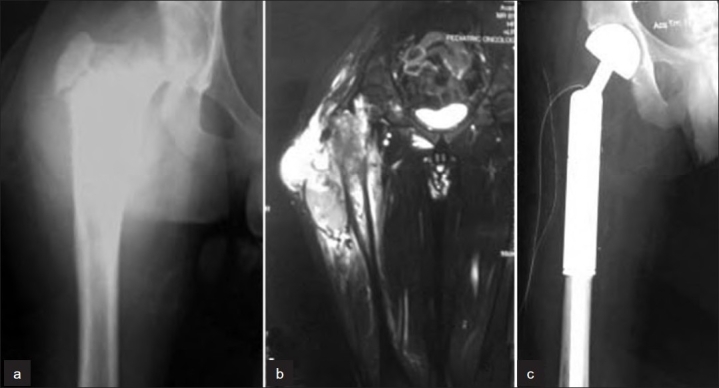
Ewing's sarcoma of the right proximal femur in a 15 yr old male, Enneking stage IIB. (a) X-ray of hip (anteroposterior view) showing the bony destruction with soft tissue mass. T2W1 saggital of hip (b) showing the intramedullary extent of the proximal femur involved along with extracompartmental extension with soft tissue mass. (c) Postoperative radiograph of hip (anteroposterior view) depicting wide resection and reconstruction with modular prosthesis
